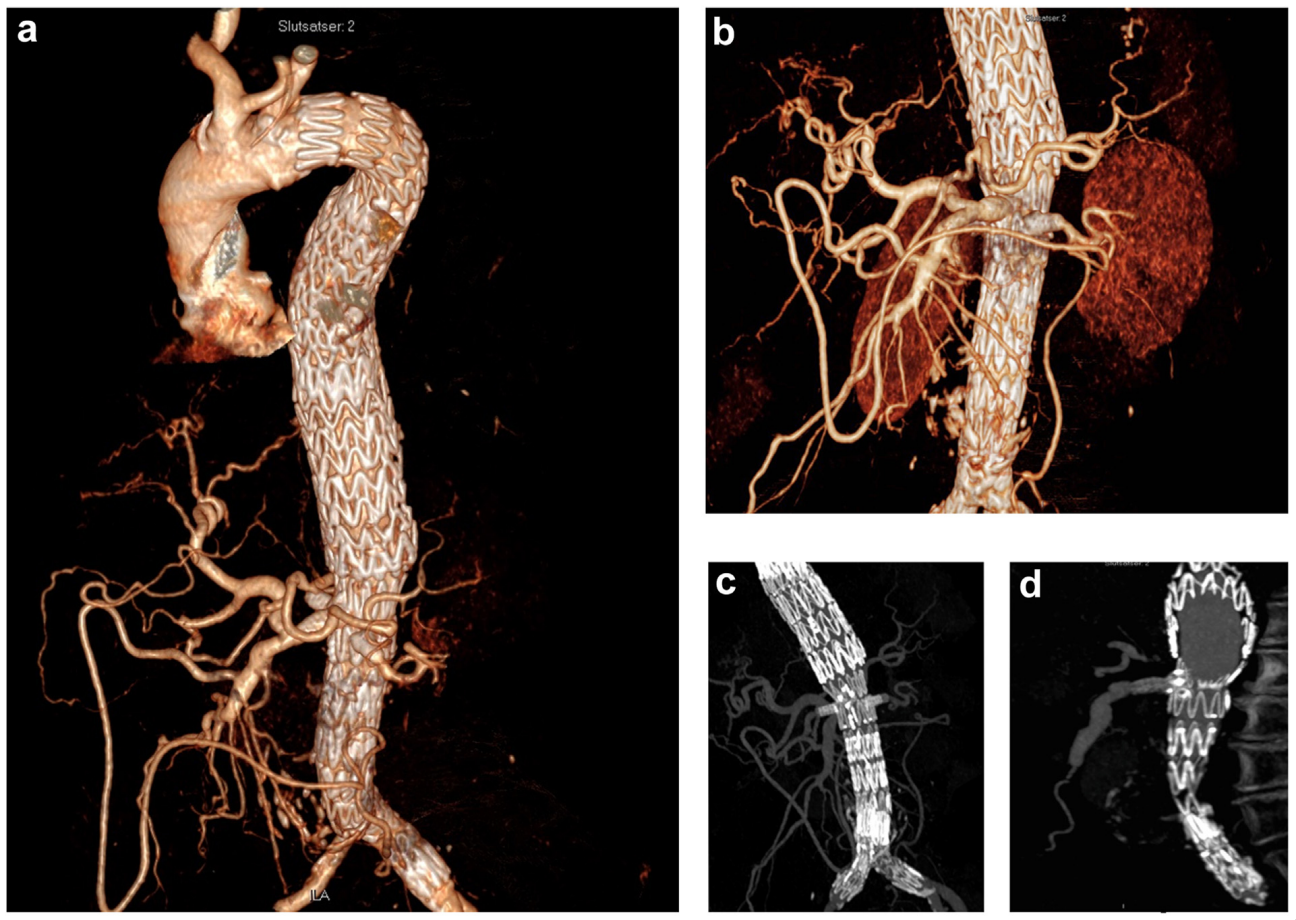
Six-month follow-up computed tomography scans. a, Three-dimensional reconstruction of computed tomography scan demonstrating the aortic metal jacket and stents to the superior mesenteric artery (SMA) and renal arteries. b, Three-dimensional reconstruction of computed tomography scan demonstrating the visceral segment. c, Coronal view of computed tomography angiogram showing patent renal artery stents. d, Sagittal view of computed tomography angiogram showing a patent SMA stent.
